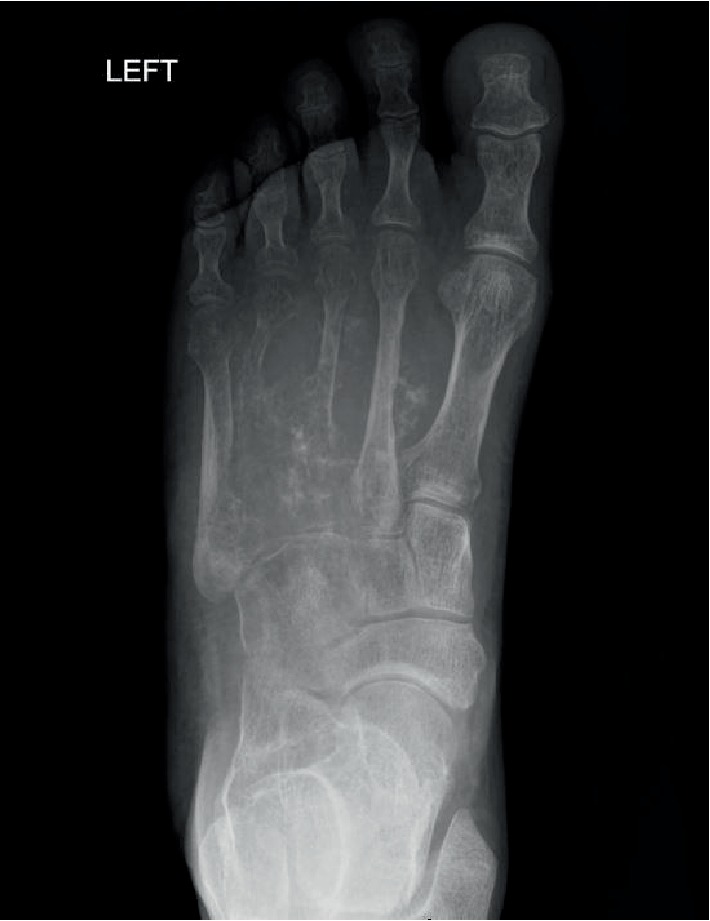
Case 2: anteroposterior left foot radiograph showing a lytic and destructive mass centered on the 4th metatarsal, with destructive extension to the 3rd metatarsal and adjacent cuneiform bones. Punctate calcifications consistent with foci of mineralization can be seen throughout the lesion.
